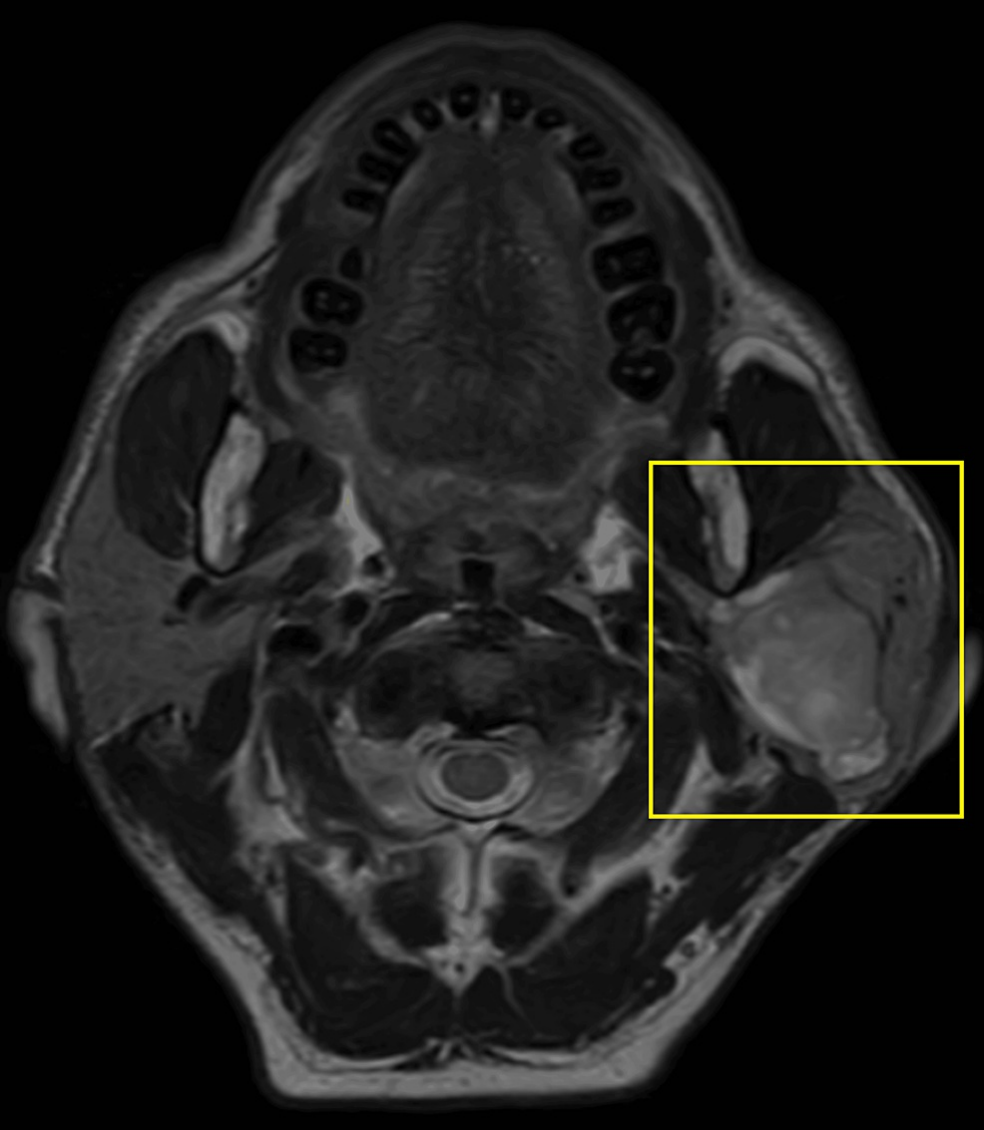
T2WI axial MRI image Highlighted area shows the physical presentation of parotid gland tumor T2WI, T2-weighted image
